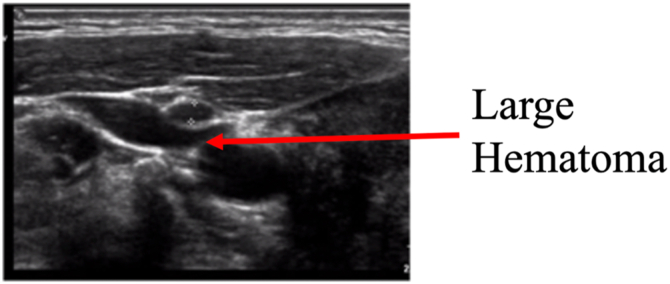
Ultrasound image obtained 2 weeks after fine-needle aspiration in 2017, showing a heterogeneous hypoechoic area (3.3 × 2.2 × 1.3 cm) within the *right* thyroid lobe, consistent with an evolving intrathyroidal hematoma.
